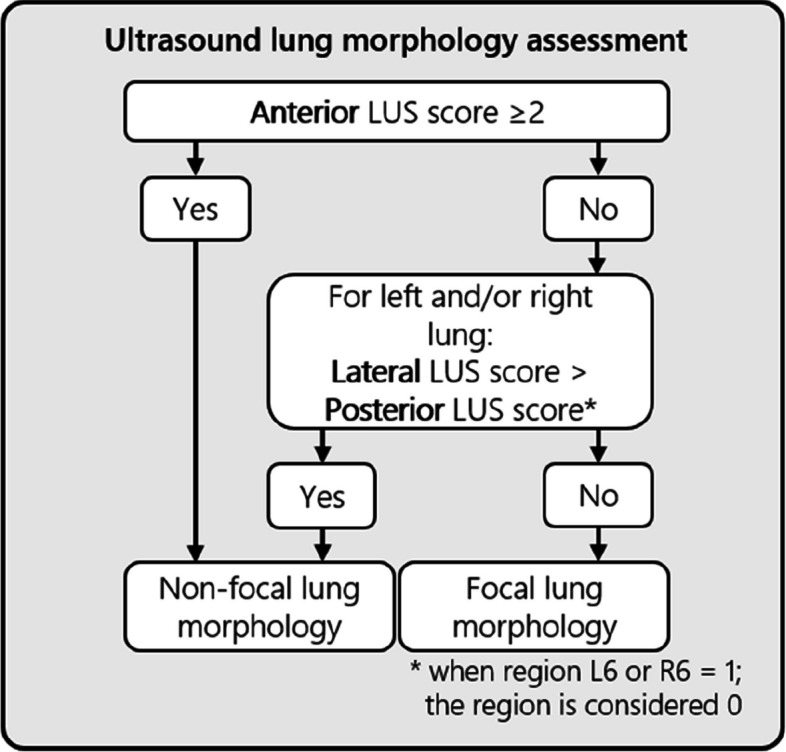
This logarithm is used to determine the lung morphology by ultrasound of eligible patients. The scores are LUS aeration scores explained in supplement 1 based on a 12-region LUS exam. *LUS* lung ultrasound
